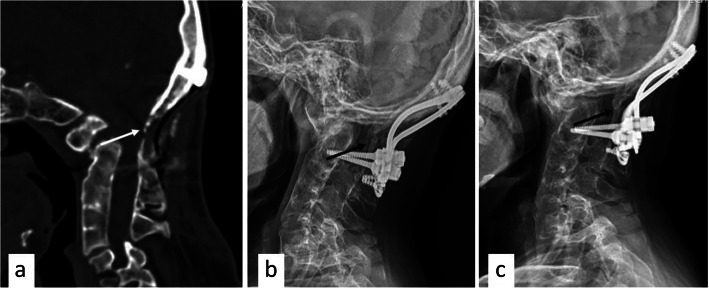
Images at follow-up. a Four months after revision surgery, CT showed the bone graft between the occipital and axis had fusion signs (white arrow). b, c Half a year and 1 year after revision surgery, X-ray confirmed a solid fusion was achieved and the internal fixation was stable (black arrow)
